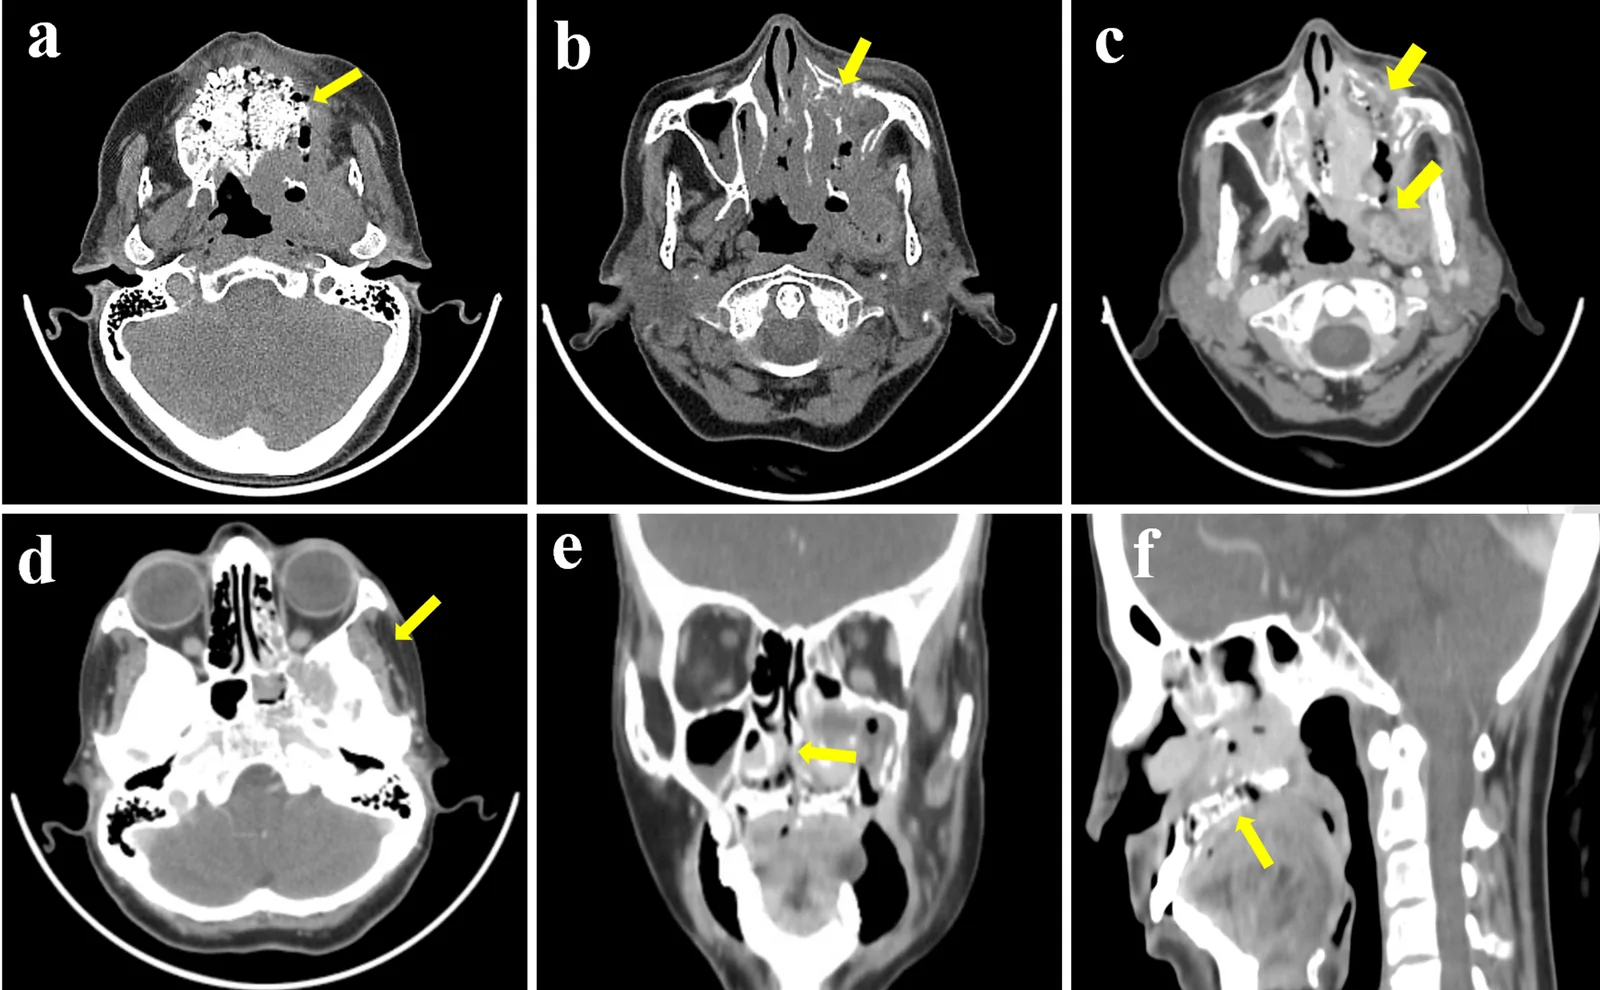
CECT of the head and neck. Axial CECT image demonstrating (a) an ill-defined enhancing soft-tissue lesion centered in the left maxilla with osteolytic destruction of the hard palate and maxillary alveolar process (yellow arrow), (b) complete opacification of the left maxillary sinus with destruction of the anterior, medial, lateral, and posterior sinus walls (yellow arrow), (c) extension of the lesion into the left nasal cavity with destruction of the lateral nasal wall and adjacent maxillary structures (yellow arrows), and (d) destruction of the left zygomaticomaxillary buttress with extension into the adjacent facial soft tissues (yellow arrow). (e) Coronal CECT image demonstrating erosion of the hard palate and left lateral nasal wall, with extension across the midline to involve the medial wall of the contralateral maxillary sinus (yellow arrow). (f) Sagittal CECT image demonstrating posterior extension of the lesion with destruction of the posterior wall of the maxillary sinus and involvement of the pterygopalatine region and infratemporal fossa (yellow arrow). CECT: contrast-enhanced computed tomography.
